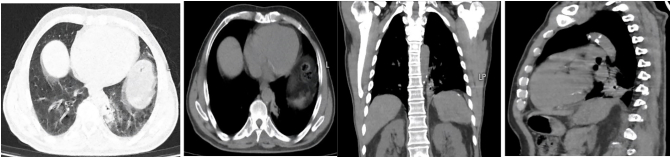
Decrease in size of cavitary lesion and peripheral consolidation after four months in lung CT scan.
